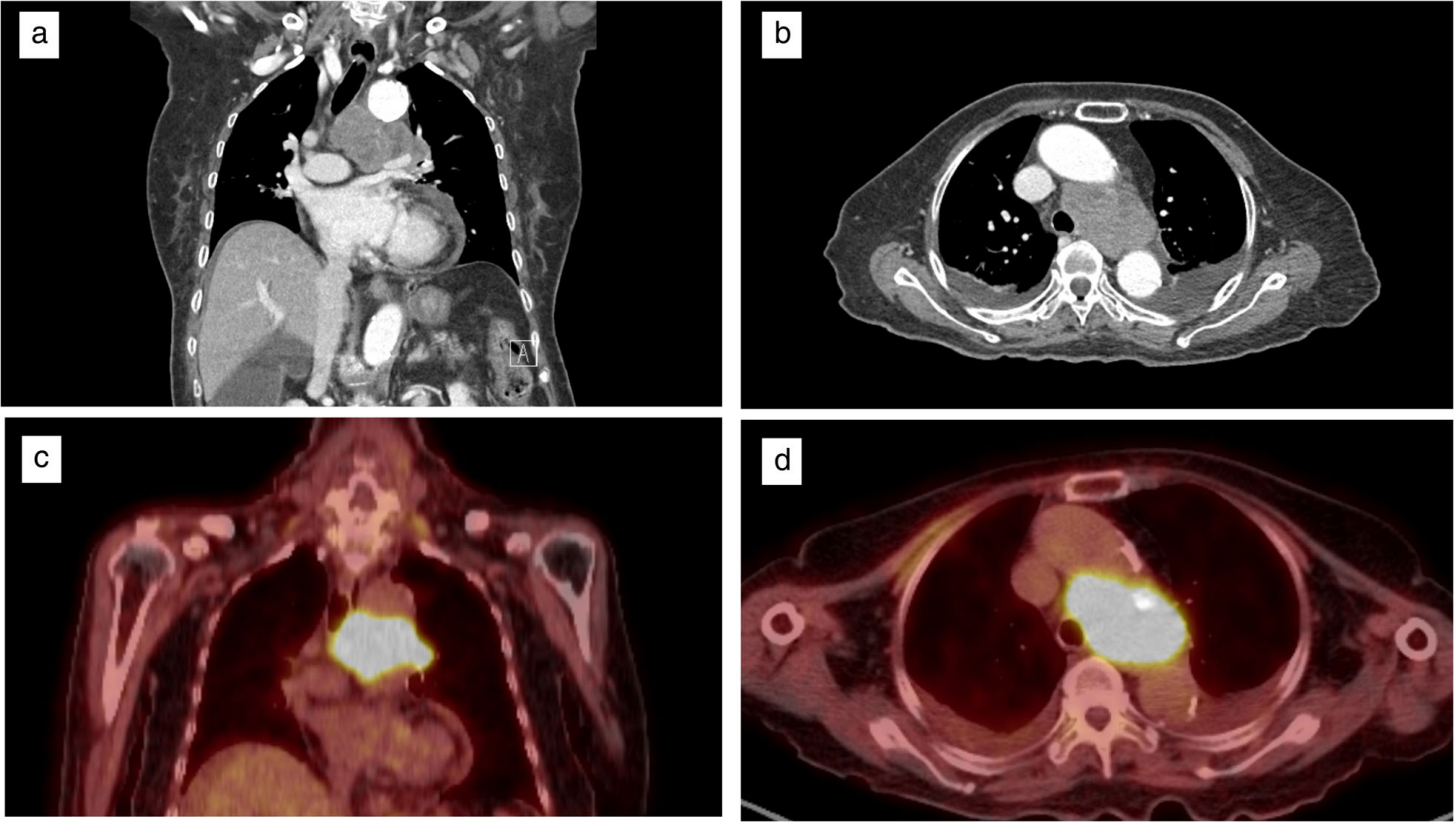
(a) Contrast‐enhanced computed tomography (CT) revealed a bulky lymphadenopathy in the left lower paratracheal area compressing the lower trachea and left main pulmonary artery. (b) On an axial section of the enhanced CT scan, a mediastinal mass compressing the left main bronchus was observed. (c), (d) 18F‐fluorodeoxyglucose‐positron emission tomography (FDG‐PET) showed high uptake of FDG with a maximal standardized uptake value of 9.2 by the tumor
